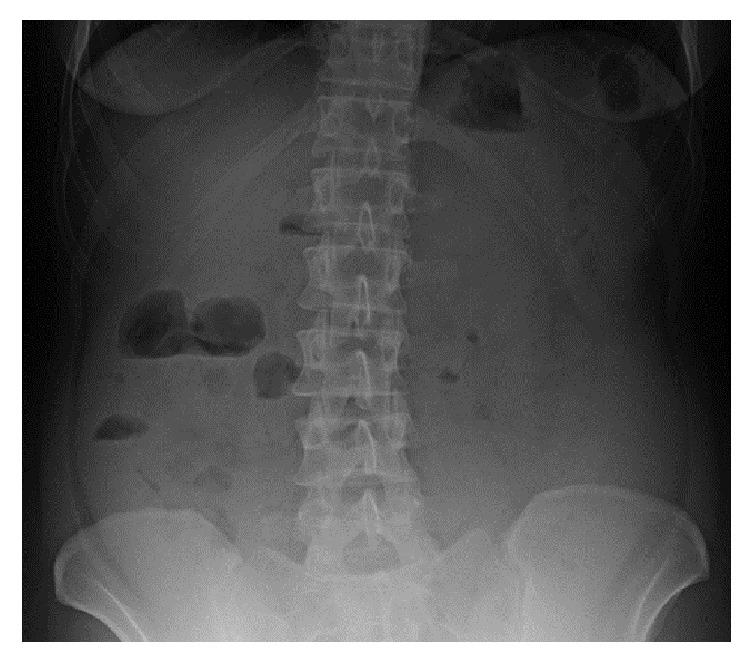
X-ray showing levels in the upper right quadrant of the abdomen.
